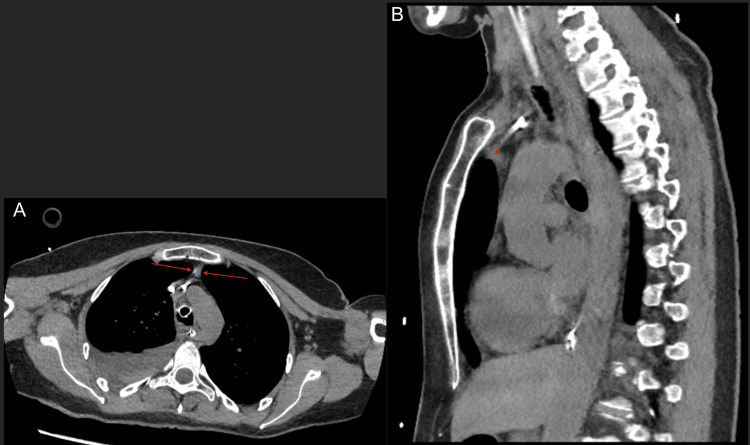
Thoracic CT scan showing the mediastinal space Left image (A) shows an axial view of the thoracic cavity with normal lung parenchyma, associated with moderate right pleural effusion and an anterior mediastinal space containing a small, non-specific ovoid thickening measuring 11 × 6 × 11 mm (red arrows; 30 Hounsfield units (HU)), possibly corresponding to residual thymic tissue or a thymic lesion. Right image (B) shows a sagittal view of the same thoracic cavity and the same non-specific ovoid thickening (red stars).
